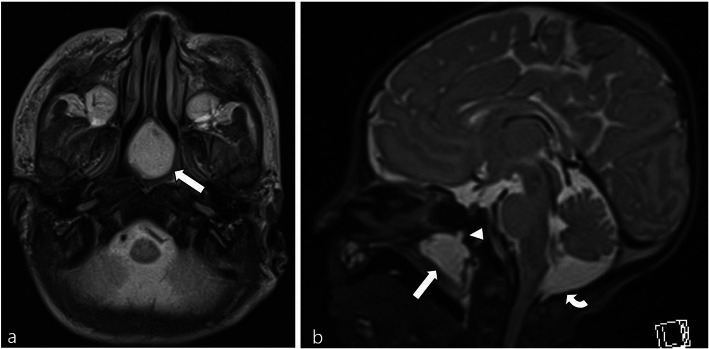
Precontrast magnetic resonance imaging of the brain. a An axial T2-weighted image and b a sagittal T2-weighed image showing a cystic mass at the choana and nasopharynx causing airway obstruction (arrows). Note a 4-mm fistula-like structure in the sphenoid bone (arrowhead). There was no discernible communication with intracranial structures. Further, a mega cisterna magna was incidentally detected (curved arrow)
